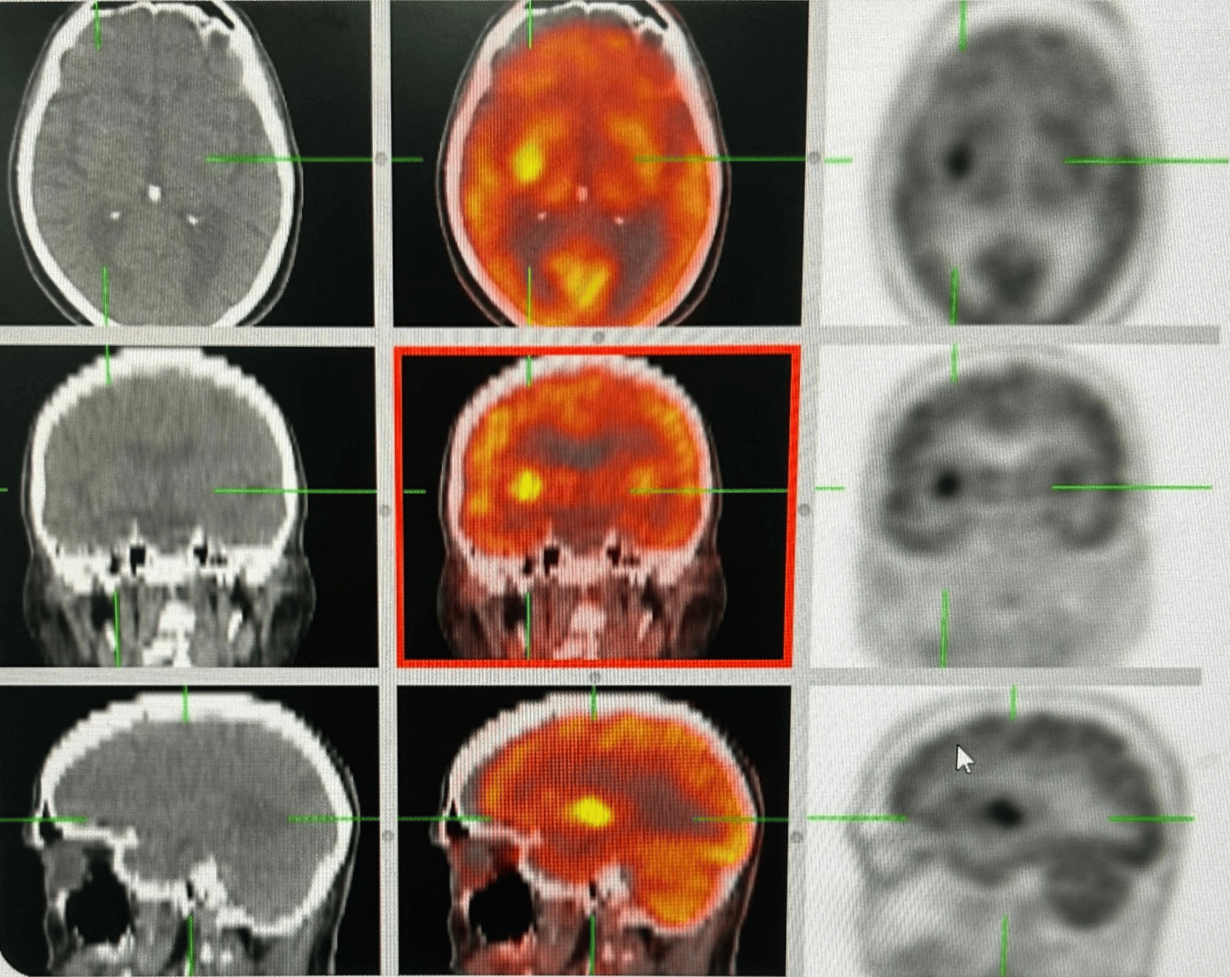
PET/CT demonstrating a new hypermetabolic focus in the right basal ganglia, compatible with recent disease activity.
